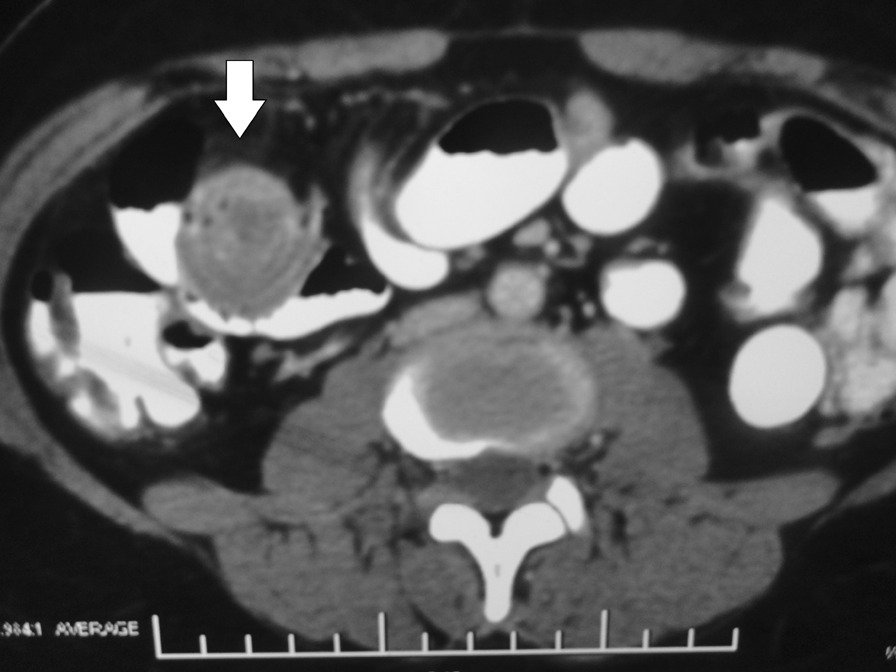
Computed tomography image (transverse plane) showing whorled pattern (arrow) suggestive of intussusception
